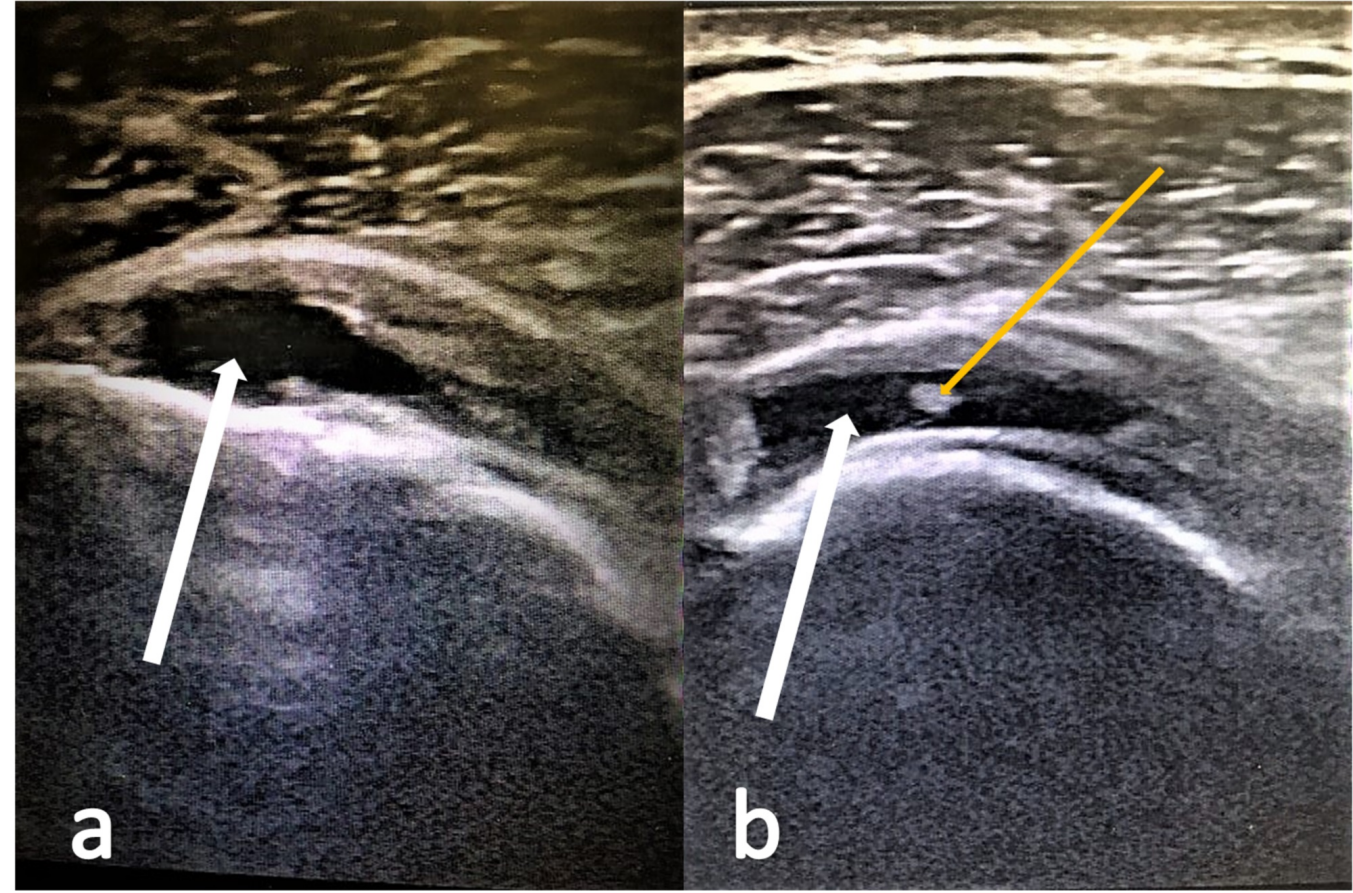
Supraspinatus tears depicted in US. a. The white arrow points to a full-thickness supraspinatus tear. b. The white arrow points to a full-thickness supraspinatus tear. The yellow arrow points to an intra-tendinous inflammation of the supraspinatus tendon. US, ultrasonography.
